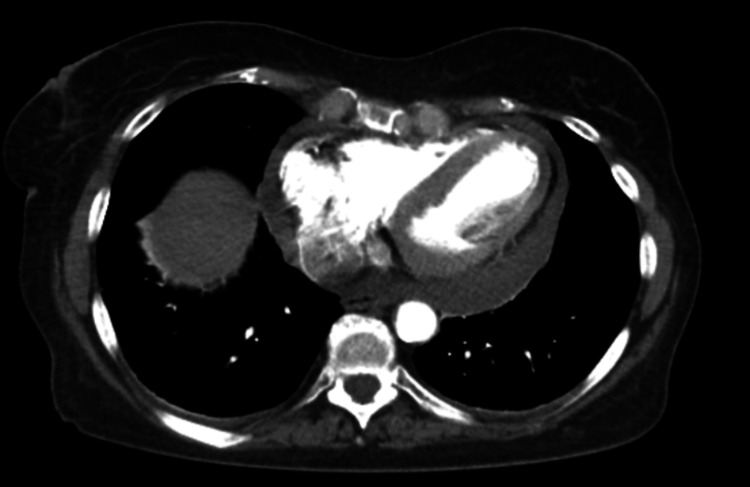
Axial CT Angiography of the Chest With Intravenous Contrast The CT shows moderate pericardial effusion. Pulmonary arteries are free of emboli, with no pleural effusions. No pathologically enlarged lymph nodes are observed. No thoracic aortic aneurysm or dissection is present. The lung parenchyma shows no consolidation or ground-glass opacities. CT: computed tomography.
